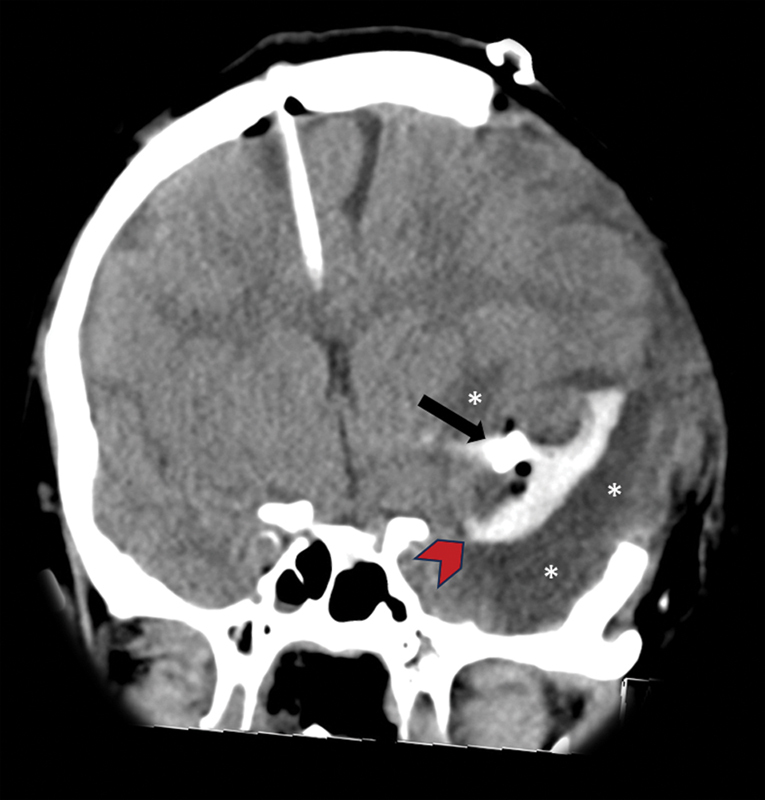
Immediate post operative CT head showing left decompressive craniectomy, right frontal ventriculostomy, aneurysm clipping (black arrow), and partial left sylvian fissure subpial hematoma evacuation (red arrowhead). Note is made for significant edematous changes along the left temporal and insular lobes (white asterisks).
